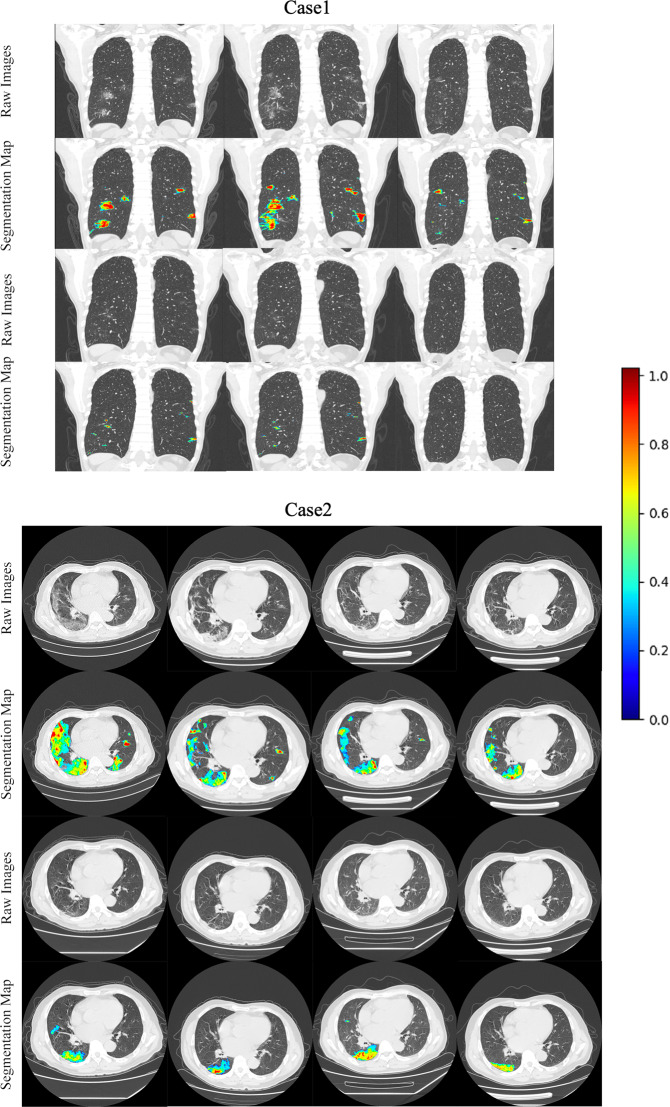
Two case studies with external longitudinal CT scans relying on dense scoremaps of lesion regions for lesion burden estimation. The raw images are shown accompanied with dense prediction scoremaps with the probability color bar. The CT images are chronologically ordered from left to right, top to down, in accordance to the scanning date as shown in the following Fig. 6.
